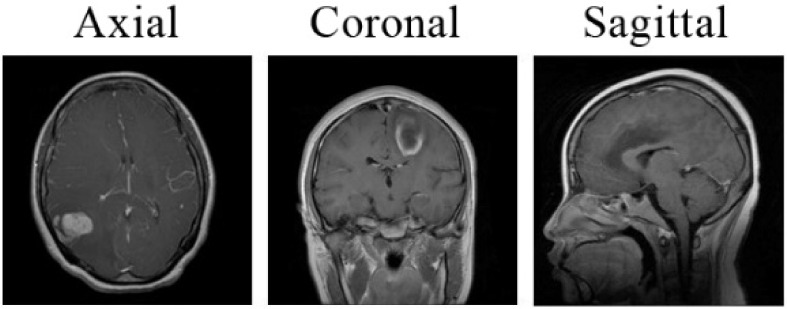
Representation of the three different planes of the MRI images.
